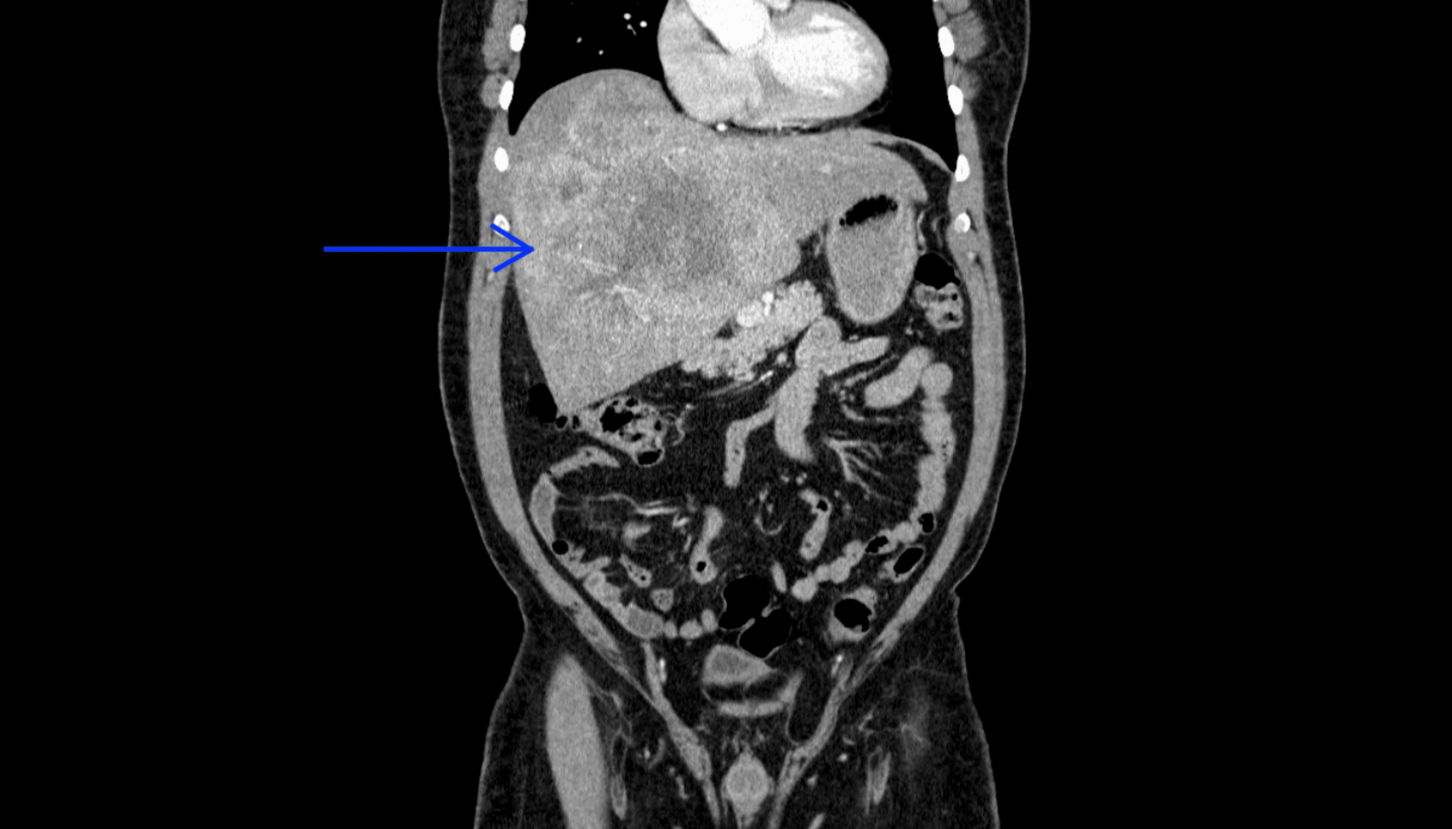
The CT of the abdomen with contrast Seen is a very large heterogeneously enhancing mass centered in the right hepatic lobe, measuring 18.3 cm x 11 cm (blue arrow).
